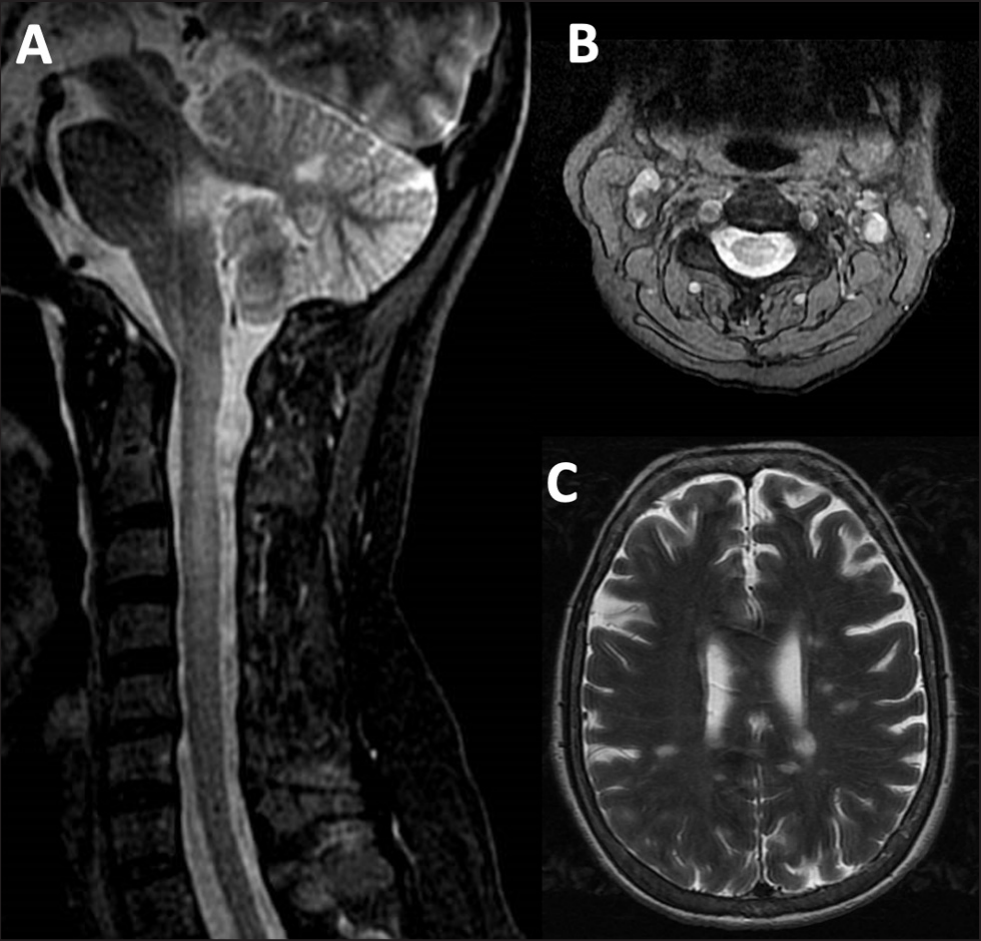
Sagittal (A) and axial (B) T2-weighted images with fat suppression highlight an area of myelitis at the C3–C4 level. Notably, hyperintensity is observed in the posterolateral region of the spinal cord. Additionally, axial T2-weighted imaging further reveals characteristic demyelinating lesions (C).
